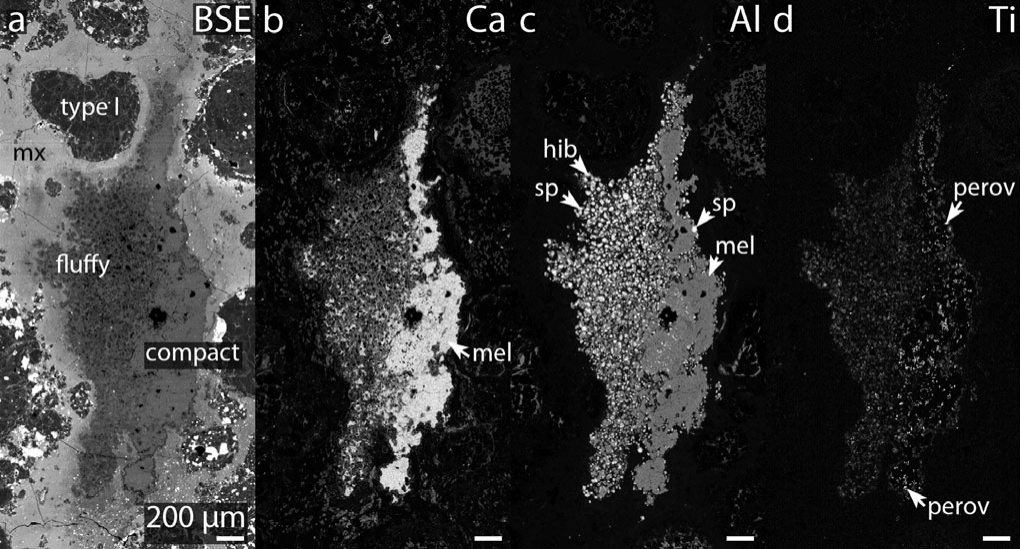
a) BSE image of a Ca-Al-rich inclusion in RBT 04133,8, and X-ray element maps of b) Ca, c) Al, and d) Ti. The CAI has both compact and fluffy textural characteristics. All scales the same. See Fig.2 and Fig. S3a for location in the thin section. mx = matrix, mel = melilite, hib = hibonite, sp = Al,Mg,Fe-spinel, and perov = perovskite.
